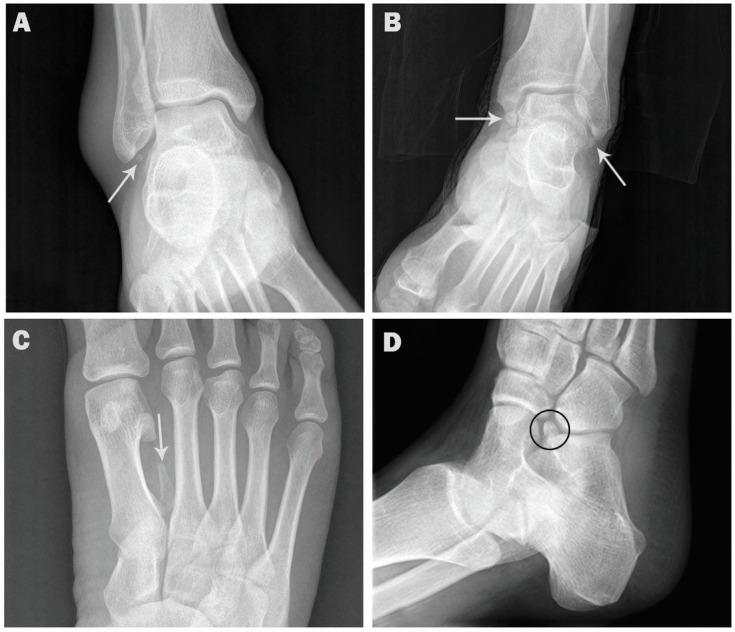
AP and oblique radiographs showing accessory ossicles. (A). AP ankle radiograph showing os subfibulare in a 53-year-old male (arrow). (B). AP ankle radiograph showing os subfibulare and os subtibiale in a 40-year-old male (arrows). (C). AP foot radiograph showing os intermetatarseum in 27-year-old female (arrow). (D). Oblique radiograph showing os calcaneus secundarius in 29-year-old female (circle).
